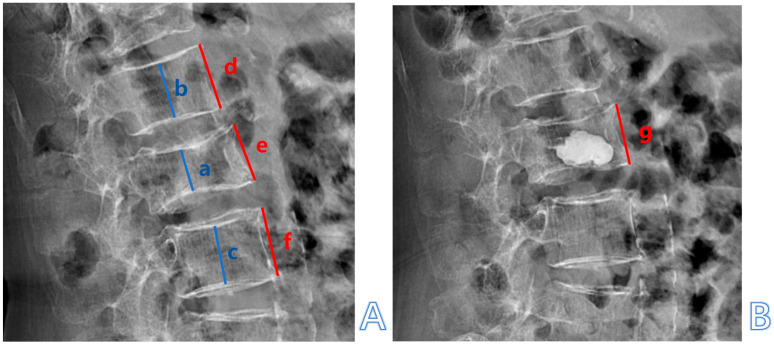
An 89-year-old female patient with an OVCF at the L2 level. (A) Preoperative lateral X-ray imaging. (B) Lateral X-ray imaging taken on the postoperative day. a. Preoperative fractured vertebral body height; b. upper adjacent vertebral body height; c. lower adjacent vertebral body height; collapse of the vertebra = 1 − 2 × a/(b + c). e. Postoperative fractured vertebral anterior margin height; d. upper adjacent vertebral anterior margin height; f. lower adjacent vertebral anterior margin height; g. preoperative fractured vertebral anterior margin height; recovery rate of vertebral anterior margin height = 2 × (e − g)/(d + f).
